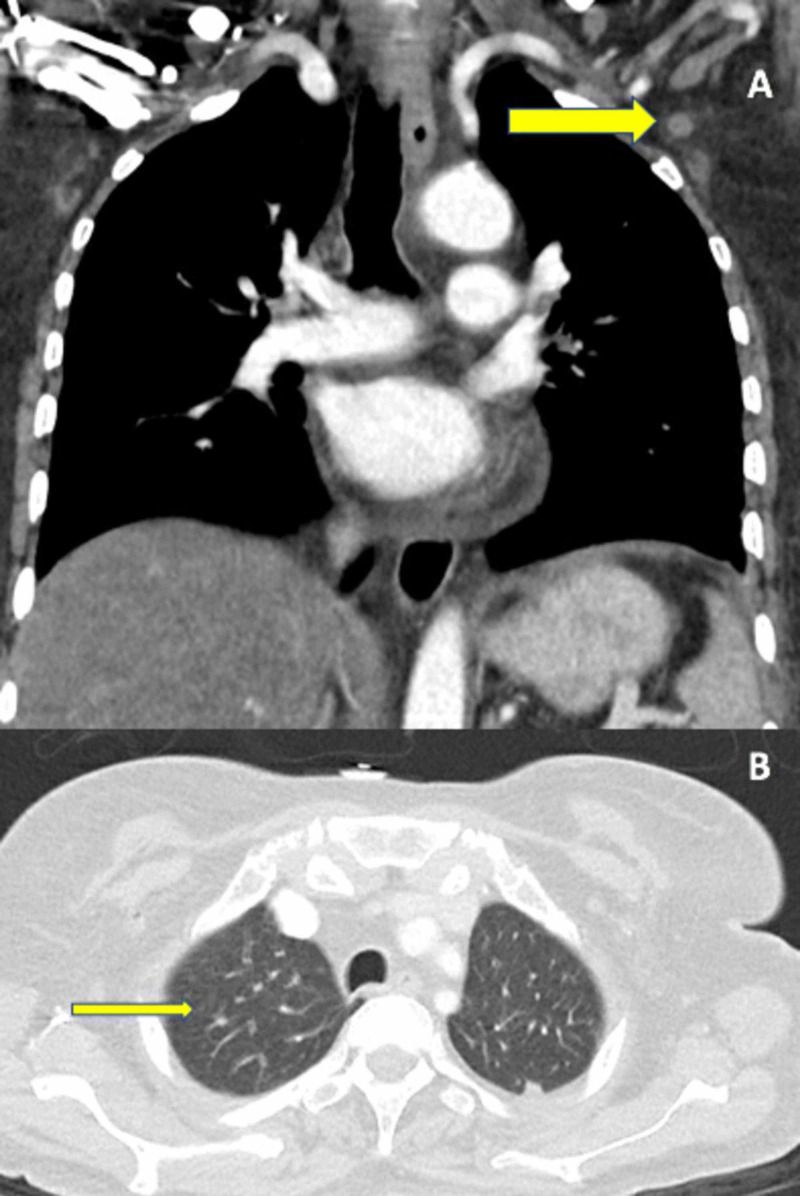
Computed Tomography (CT) Findings A: Mildly Prominent Axillary Lymph Nodes B: 5-mm Left Upper Lobe Pulmonary Nodule
